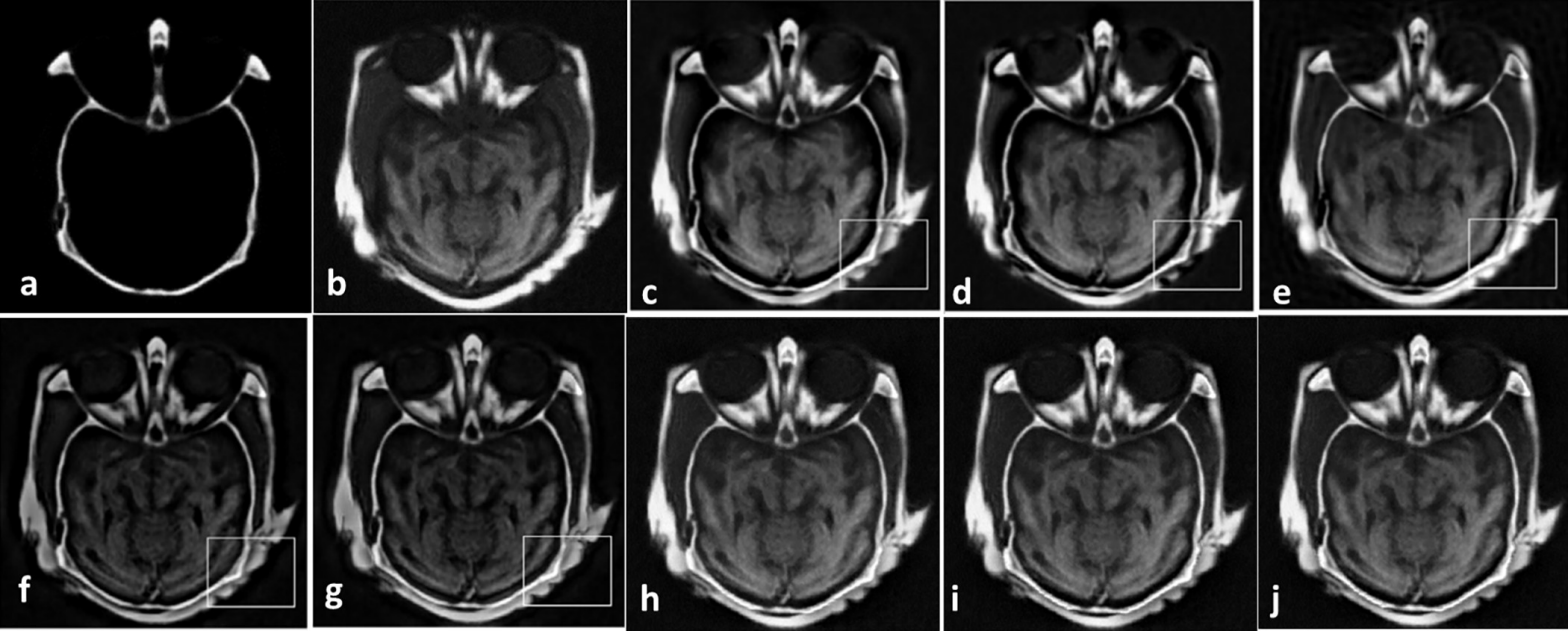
Experiment 5 [53]: Results of fusing CT and MR images of a brain. (a) CT image; (b) MR image; (c) NNSST [93]; (d) FMSAP [95]; (e) CST [97]; (f) ST-NSST [96]; (g) MFDF-NSST [53]; (h) the proposed method with PCA, (i) max, and (j) weighted averaging model, respectively.
